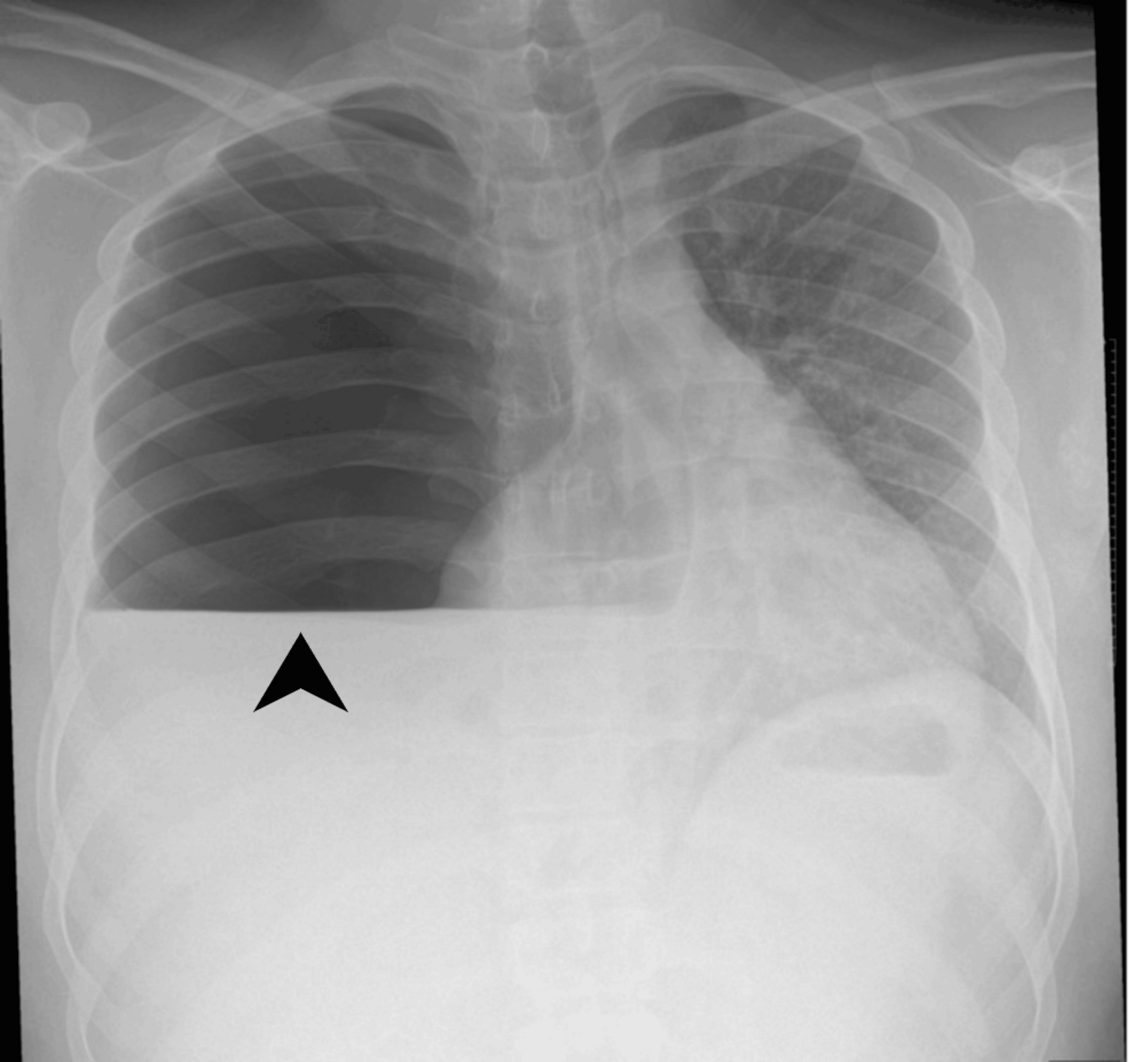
Posteroanterior chest radiograph demonstrating right-sided hydropneumothorax. There is a visible air-fluid level in the right hemithorax, characterized by a horizontal interface suggestive of concurrent pleural air and fluid (arrowhead).
